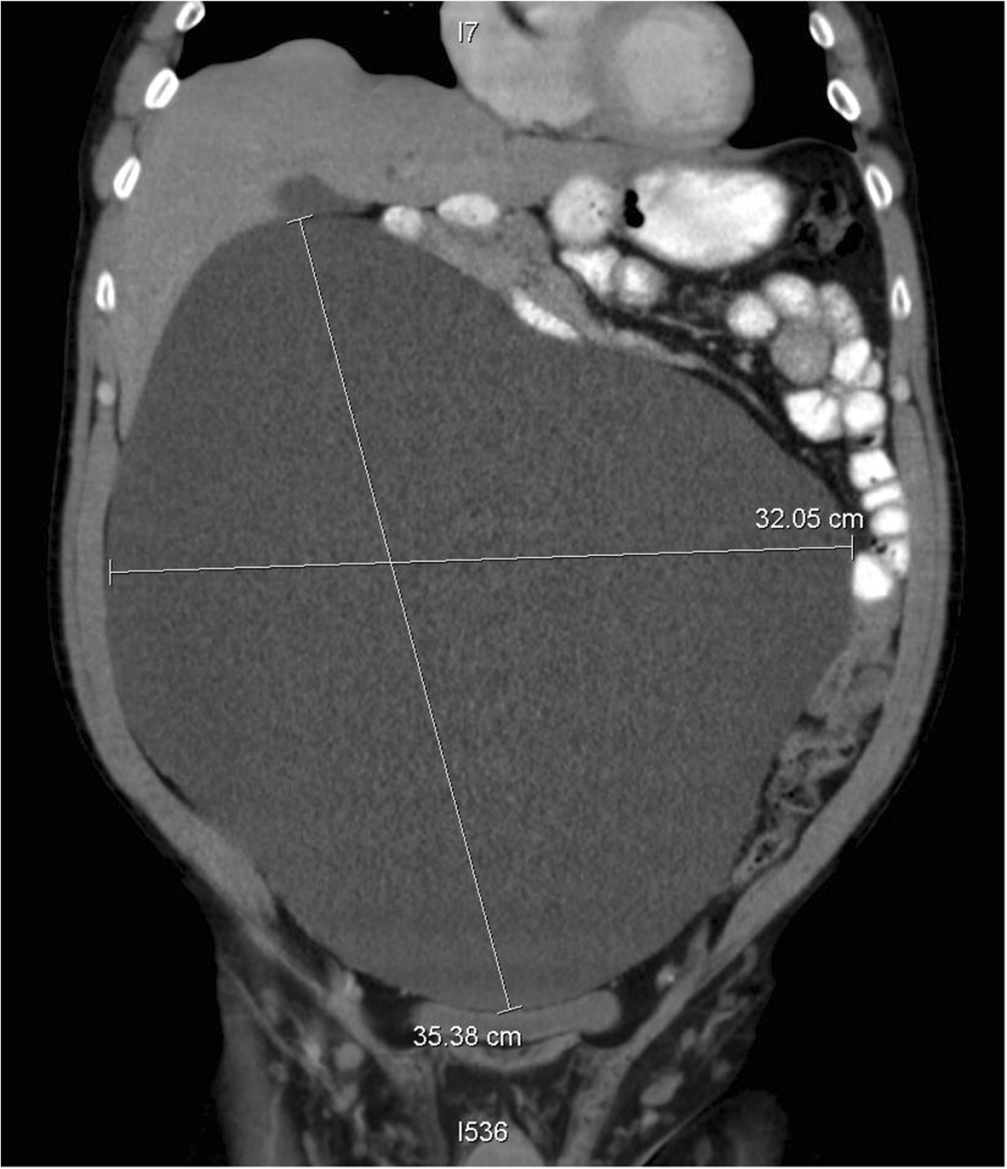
Pre-operative computer tomography (CT) scan images (axial, coronal and sagittal views of the giant cyst).
